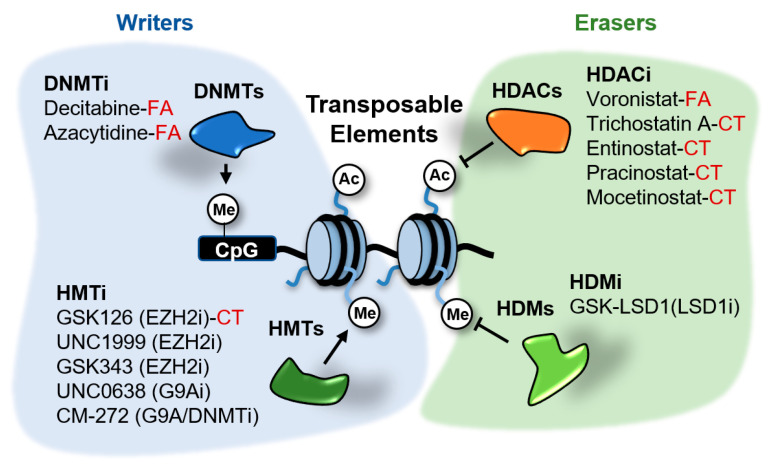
Epigenetic inhibitors described to stimulate the expression of transposable elements (TEs) in cancer cells. These inhibitors target the writers DNA methyltransferases (DNMTs) and histone methyltransferases (HMTs), and the erasers histone deacetylates (HDACs) and demethylases (HDMs). Compounds in clinical trials (CT) or FDA-approved (FA) for cancer treatment are indicated. The rest of compounds are in preclinical testing.
